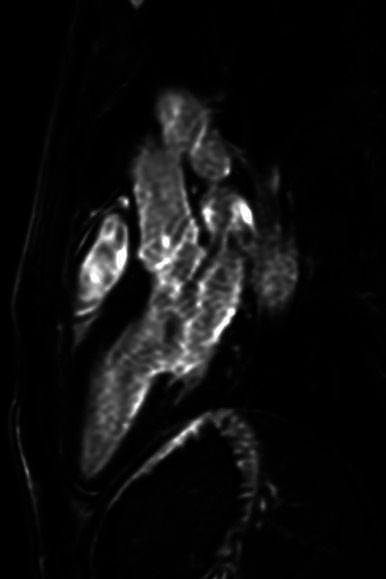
MRI image analysis. Postcontrast images: on late images, the presence of enhancement areas with lower basal distribution compatible with myocardial fibrosis.
